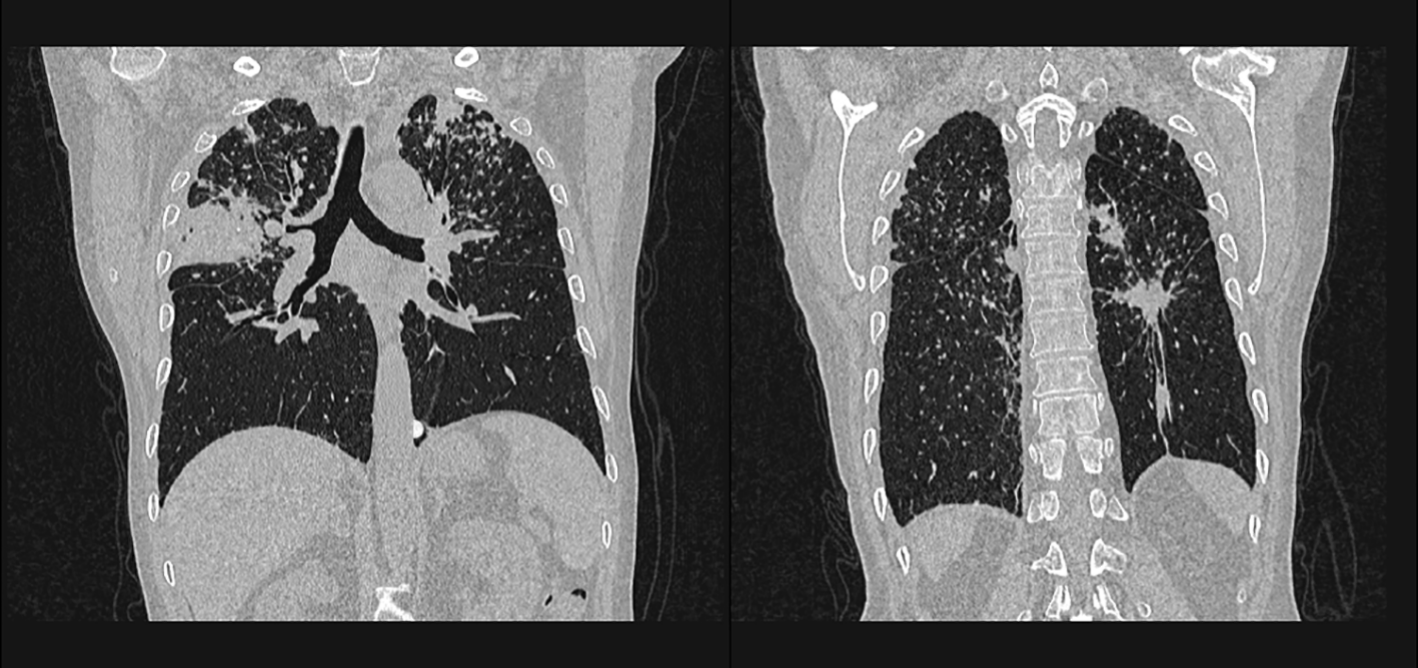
Chest CT on current admission showing the distribution of pulmonary lesions.
